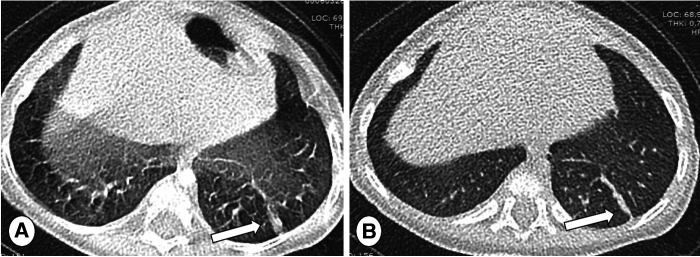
Axial CT of 5 months old patients with CF, thickness 0.7 mm, scanned in single source-mode with pitch 1.2 (A) and repeated in double source-mode with pitch 3.2 (B). Note that respiratory artifacts are absent using an higher pitch acquisition (B), providing a high image quality and facilitating the detection of atelectasis in the left lower lobe (arrows).
